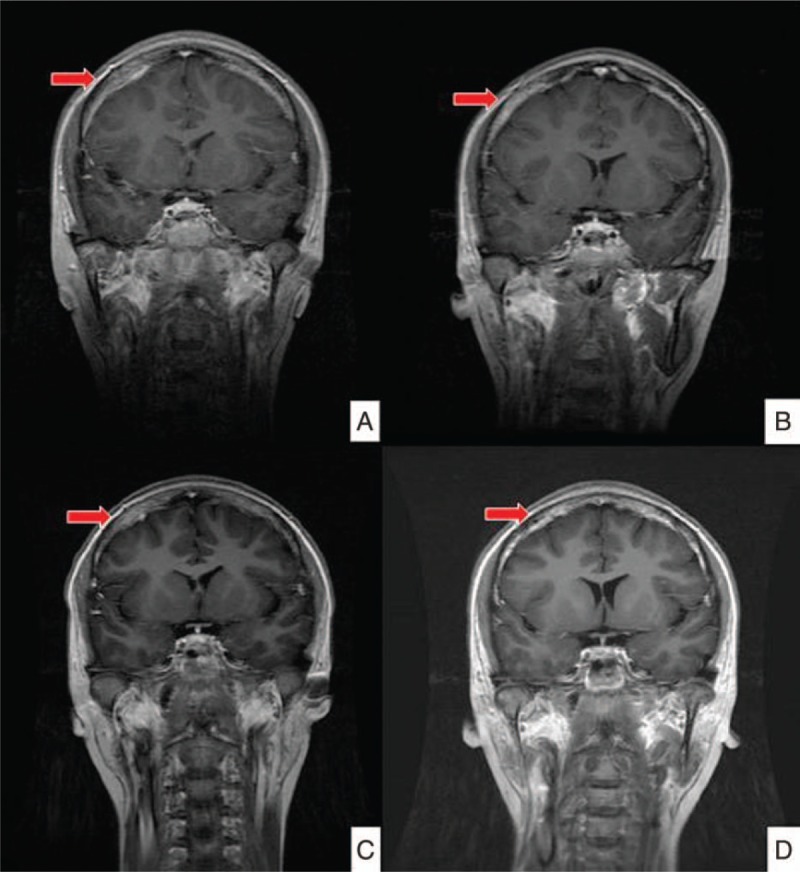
Serial images of brain magnetic resonance imaging. (A) Newly found brain metastasis in 2013. (B) Improvement of brain metastasis after chemotherapy. (C) Residual brain metastasis in 2014. (D) Follow-up image in 2015 after whole brain radiotherapy in 2014 (red arrows indicate the lesions).
